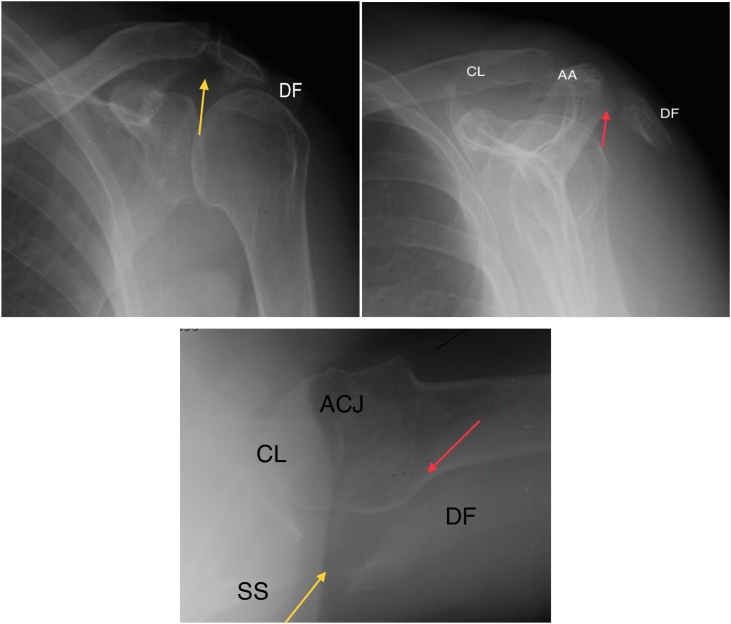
Left shoulder x-rays. a) AP view, yellow arrow: displaced fracture of lateral angle of the scapular spine; b) outlet view, red arrow: displaced acromion fracture; c) axillary view, yellow and red arrows: the same as figure a and b; CL- clavicle; AA- anterior acromion; DF- displaced fragment; SS- spine of the scapula; ACJ- acromioclavicular joint.
